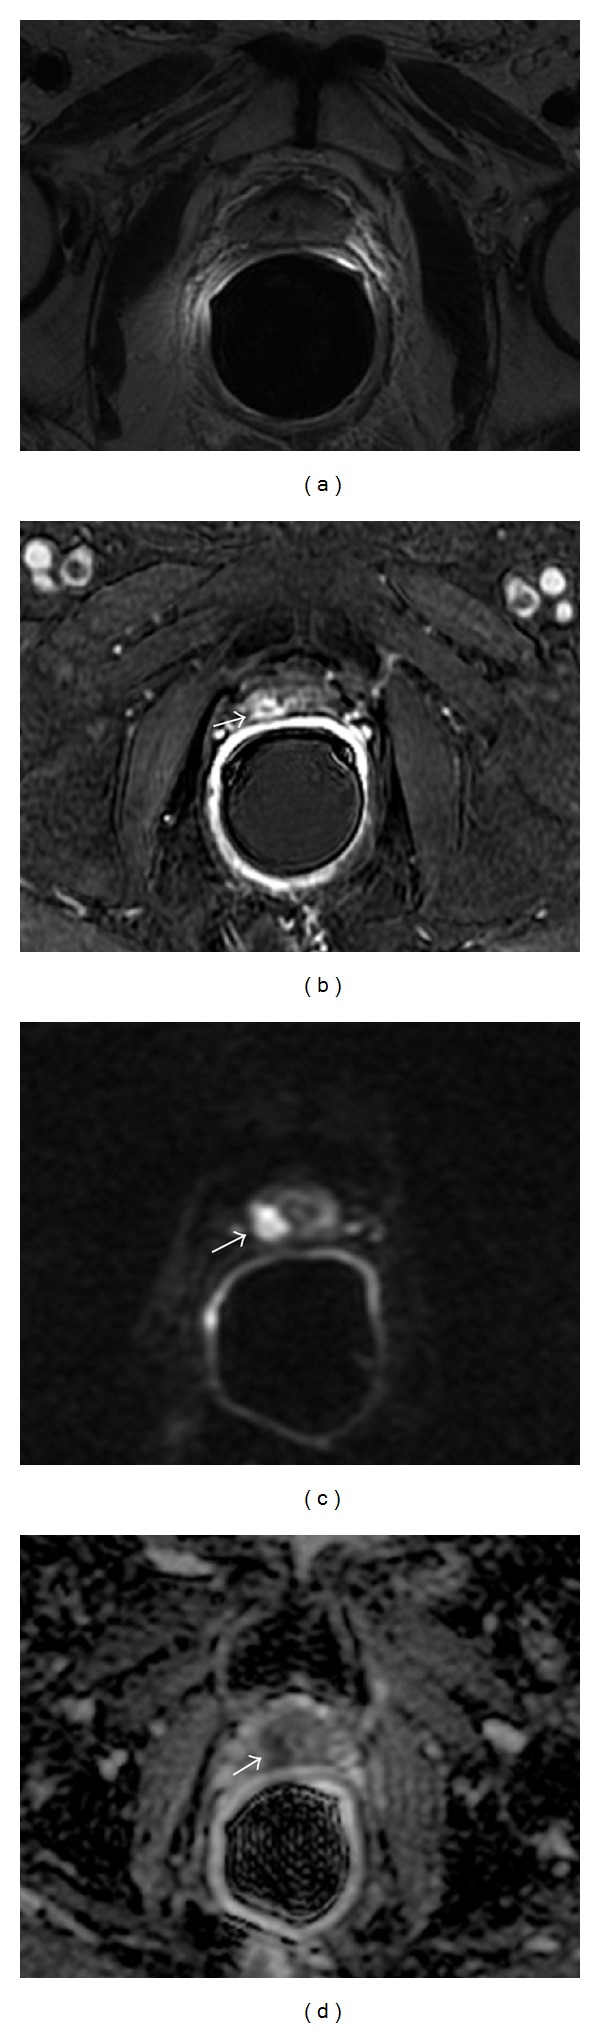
Multiparametric-MR images obtained in a 69-year-old patient with TRUS-guided biopsy-proved PCa (Gleason score, 3 + 4) in the right apex-midgland after 14 months after external beam radiation therapy. (a) Axial T2-weighted fast spin-echo image shows shrinkage of the prostate gland which appears diffusely hypointense because of radiation induced atrophy and fibrosis. No suspicious foci are seen. (b) Axial gradient-echo T1-weighted subtracted image showing a remarkable nodular enhancement at the right apex-midgland (white arrow). (c) Axial native DWI image at *b* value = 3,000 s/mm^2^ showing a focus with marked restricted diffusion (white arrow). (d) Axial ADC map reconstructed from images obtained at *b* values of 0, 500, 1,000, and 3,000 s/mm^2^ shows a dark area corresponding to the hypervascular nodule (white arrow). All these findings are consistent with local recurrent PCa.
